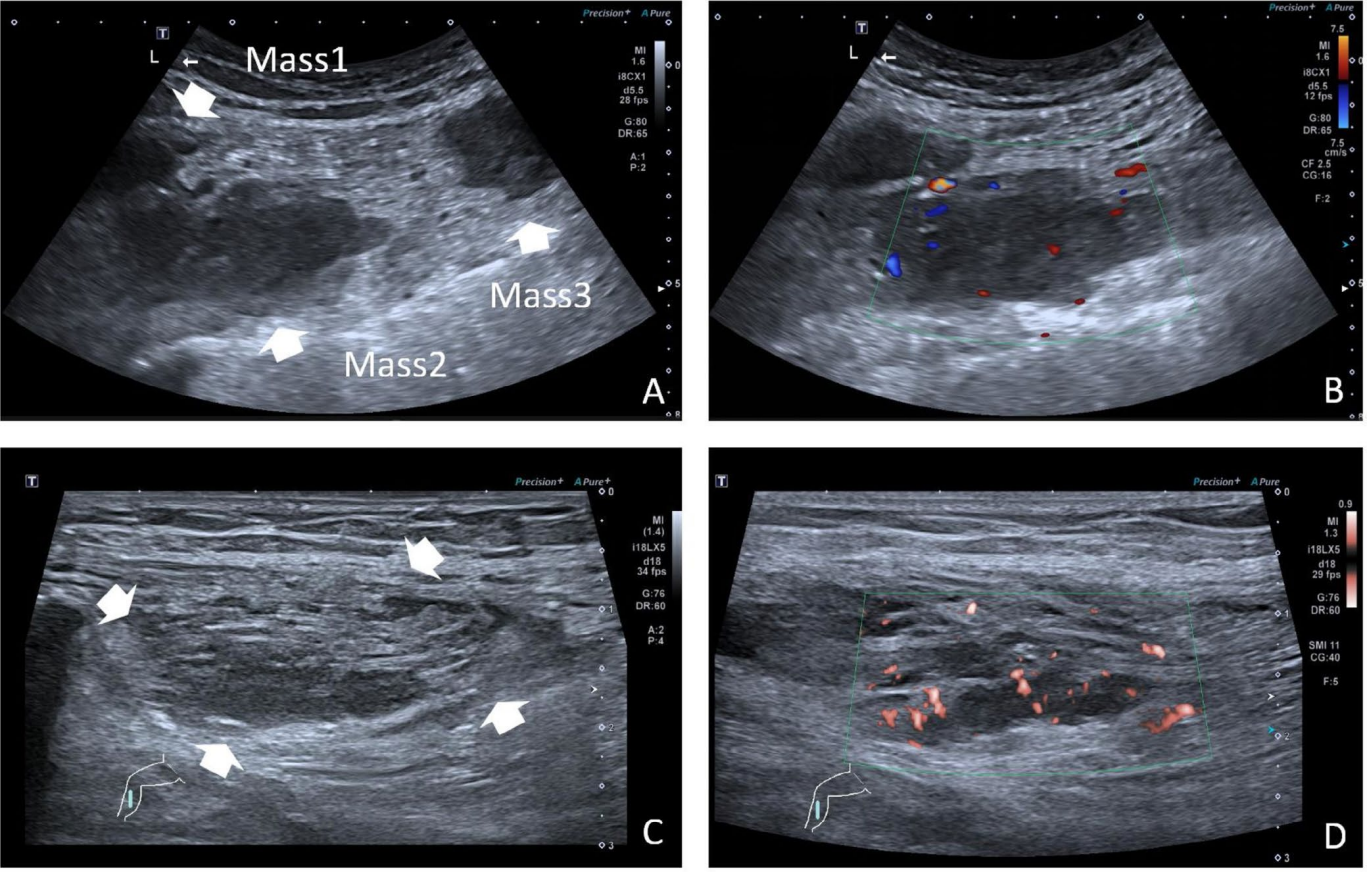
(A) Ultrasonographic image of multiple metastatic lesions in the left thigh. (B) CDFI image of metastatic lesions in the left thigh. (C) Ultrasonographic image of metastatic lesions in the right calf. (D) SMI of metastatic lesions in the right calf.
